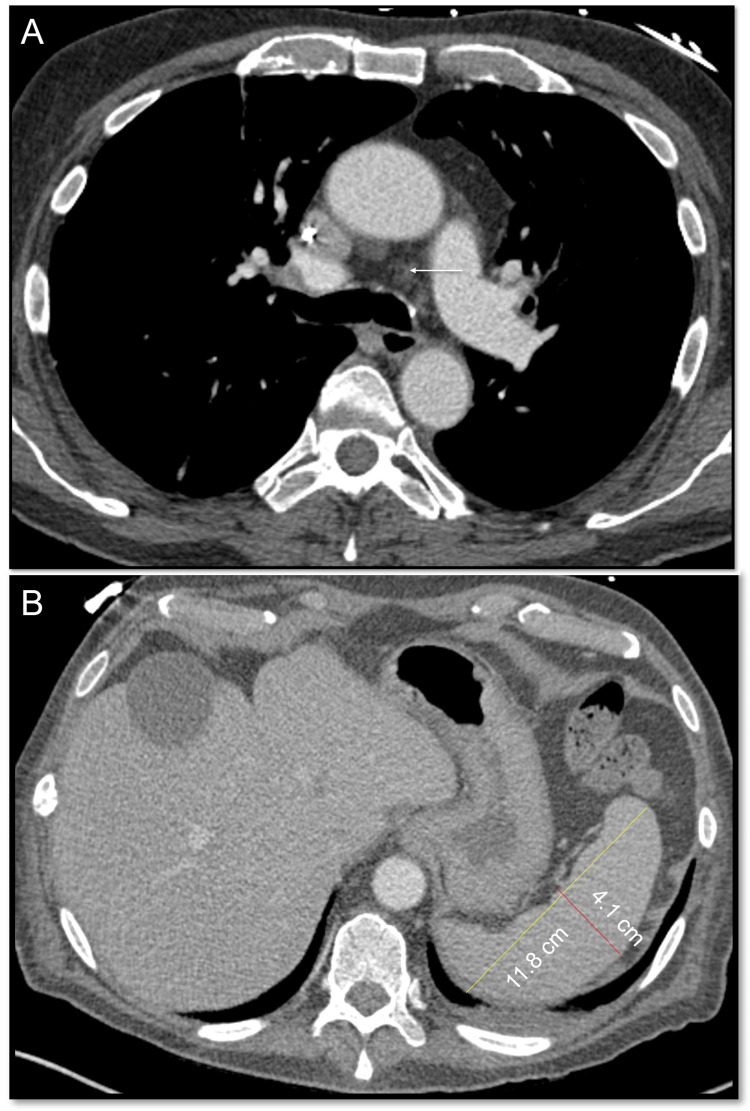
Repeat CT chest (A) Repeat CT chest shows resolution of lymphadenopathy (arrow); (B) repeat CT abdomen shows resolution of splenomegaly.
